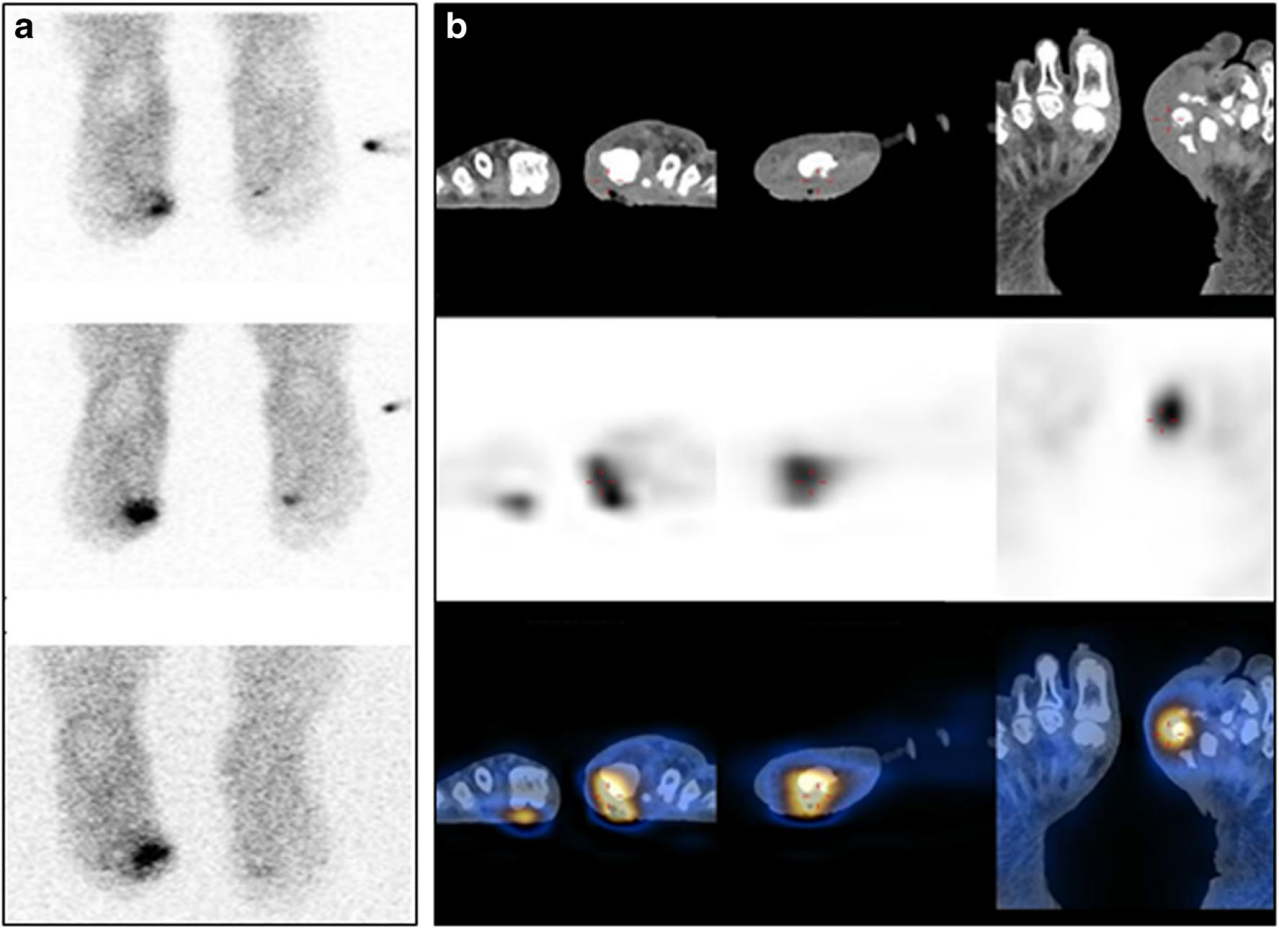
^99m^Tc-HMPAO-labelled leucocyte SPECT/CT in a patient with diabetic foot. A 43-year-old man with diabetes mellitus and an infected wound in the medial aspect of the left forefoot was referred for ^99m^Tc-HMPAO-leucocyte scintigraphy for suspected osteomyelitis. Planar scans (a) performed at 1 h (top), 4 h (center), and 24 h (bottom) after tracer injection show a focal area of uptake at the base of the first left digit, increasing in intensity in its superior aspect. SPECT/CT (b) locates this uptake to fragments of the first left metatarsal bone showing areas of cortical erosion and sclerosis, as well as to the adjacent deep wound and surrounding edematous soft tissue, consistent with osteomyelitis in addition to the soft tissue infection
